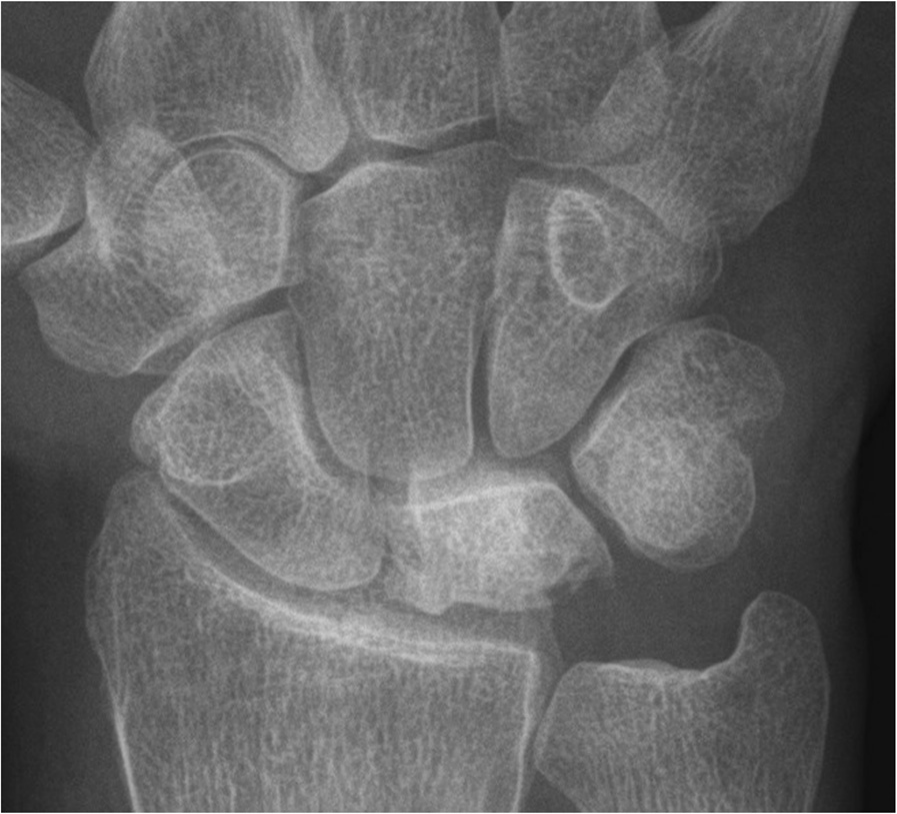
Post-operative pa x-rays of the right wrist of a 31-year-old male patient who had undergone RSO
